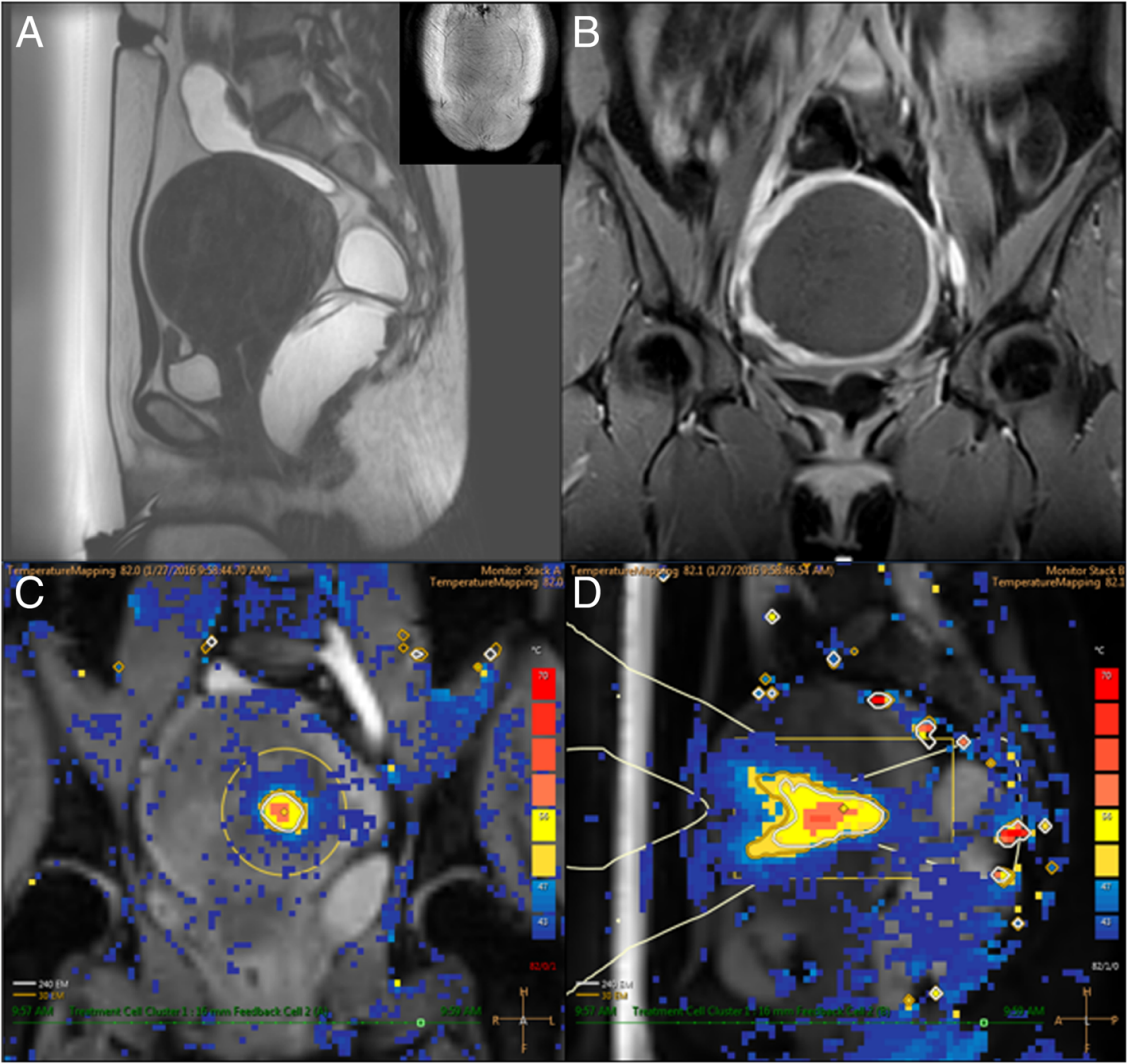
A 33-year-old woman with 10.1-cm uterine fibroid, without an abdominal scar. a Sagittal T2W planning MR image of uterine fibroid prior to MRgHIFU treatment. b CE-T1W image acquired immediately after MR-guided high-intensity focused ultrasound treatment. The NPV ratio was 100%. c, d An example of multiplane MR thermometry acquired in both coronal and sagittal planes during sonication
